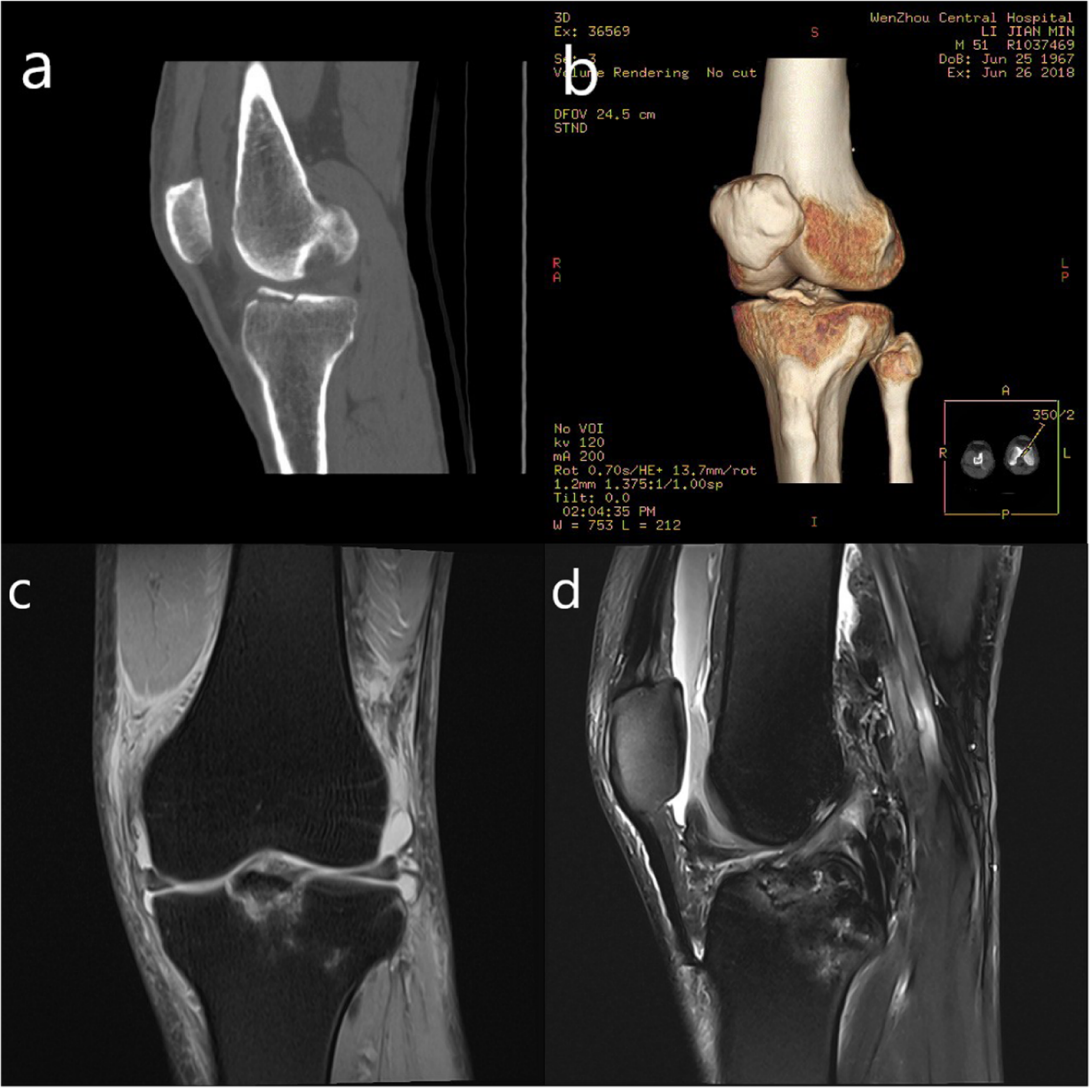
Preoperative images. a, b Preoperative computed tomography scan showing an example of a type III tibial intercondylar eminence avulsion fractures. c, d Preoperative MRI result showed a displaced (Type III) avulsion fracture of the tibial intercondylar eminence
